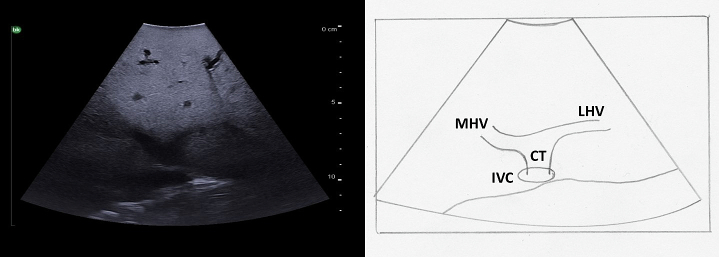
A common trunk is formed by the union of the left and middle hepatic veins before they empty into the inferior vena cava. MHV middle hepatic vein, LHV left hepatic vein, IVC inferior vena cava, CT common trunk.
